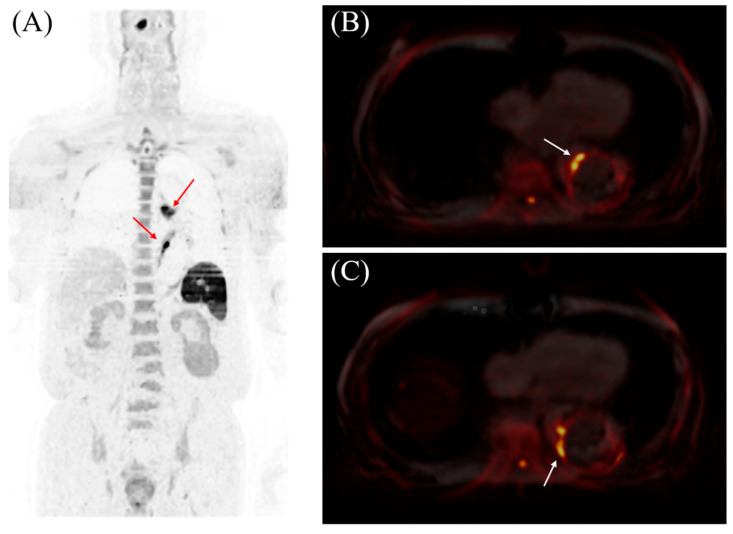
The results of magnetic resonance imaging using diffusion-weighted whole-body imaging with background body signal suppression (DWIBS). (A) Three-dimensional rendering of the DWIBS data demonstrates high signal intensity surrounding the aorta (red arrows). (B,C) Axial slices from the DWIBS dataset (original images) show hyperintensity within the aortic wall (white arrows). DWIBS, pioneered by Takahara et al. in 2004, is an advanced MRI-based technique for comprehensive whole-body cancer screening [1]. This technique employs a short τ inversion recovery echo-planar imaging sequence with free breathing for the highly sensitive detection of diffusion-restricted lesions, such as cancers and abscesses, by leveraging the movement of water molecules to create sharp contrasts between abnormal and normal structures across the entire body simultaneously [1,2,3,4]. This provides a level of convenience comparable to positron emission tomography–computed tomography (PET-CT) [3]. DWIBS offers both cost-effectiveness and the advantage of being non-invasive, as it eliminates the need for expensive radionuclides that may otherwise pose a risk of radiation exposure [3]. Additionally, DWIBS has been reported to detect inflammatory pathology, with its most common application being Takayasu aortitis [5,6]. In addition, there is a case of aortic inflammation related to giant cell arteritis detected by DWIBS [7]. In this case, high DWI signals were observed along the aortic wall, indicating such inflammation. Cardiovascular surgeons suspected that the inflammation was associated with an aortic stent ulcer, but conclusive evidence to support this was unavailable. Nevertheless, based on the previous reports and our case, we believe that DWIBS holds promise as a useful modality for detecting inflammatory lesions, particularly in the aorta.
